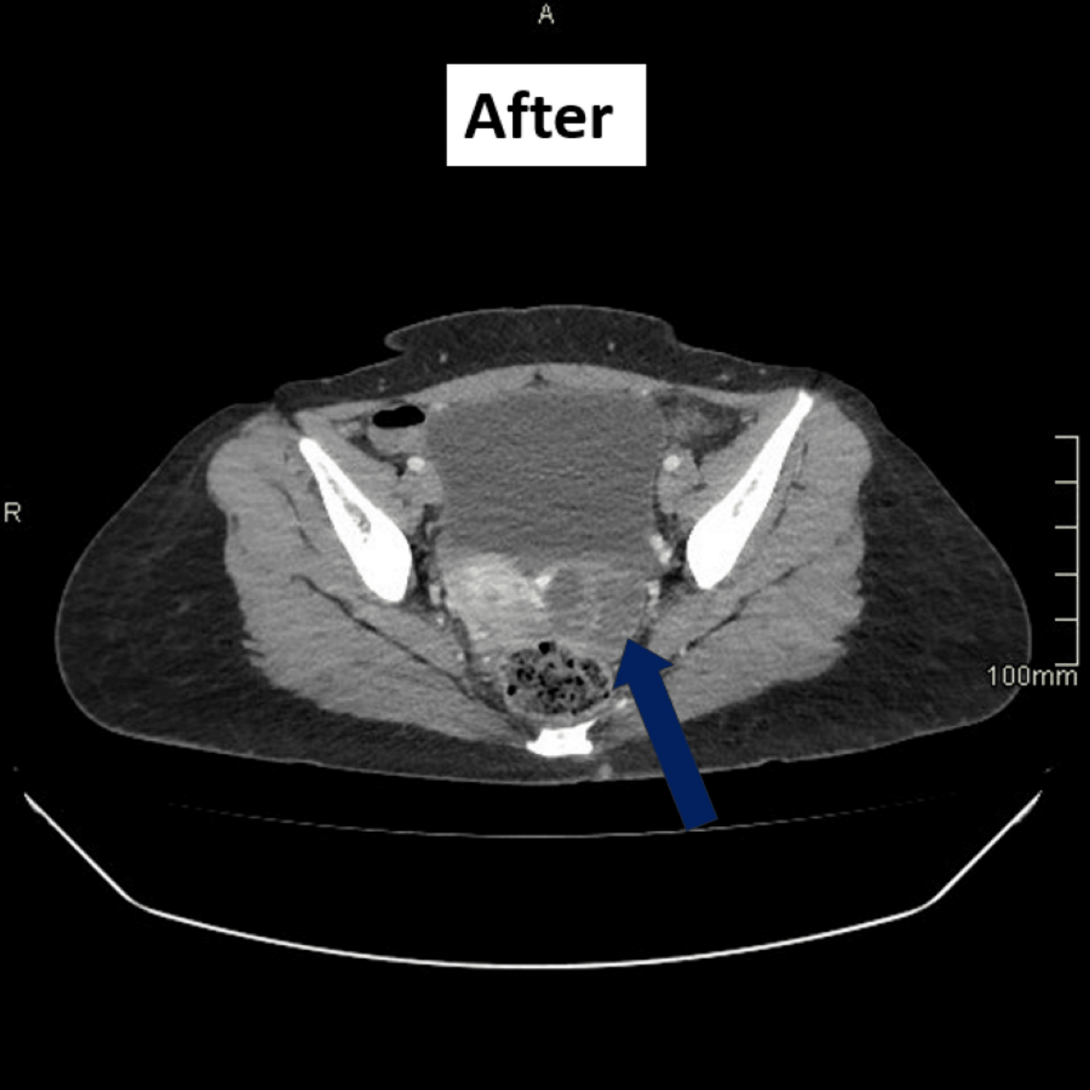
CT after initial treatment. The arrow points to the left adnexal mass, which has reduced in size after three months of treatment.
